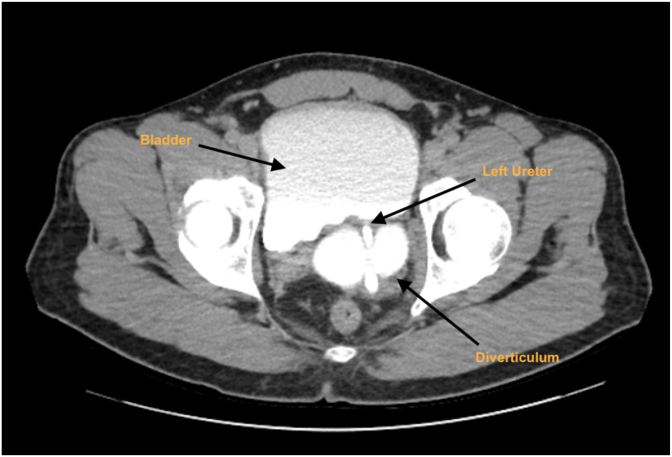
Computer tomography intravenous pyelogram (CT IVP) axial slice demonstrating direct compression of the left ureter by large bladder diverticulum.
